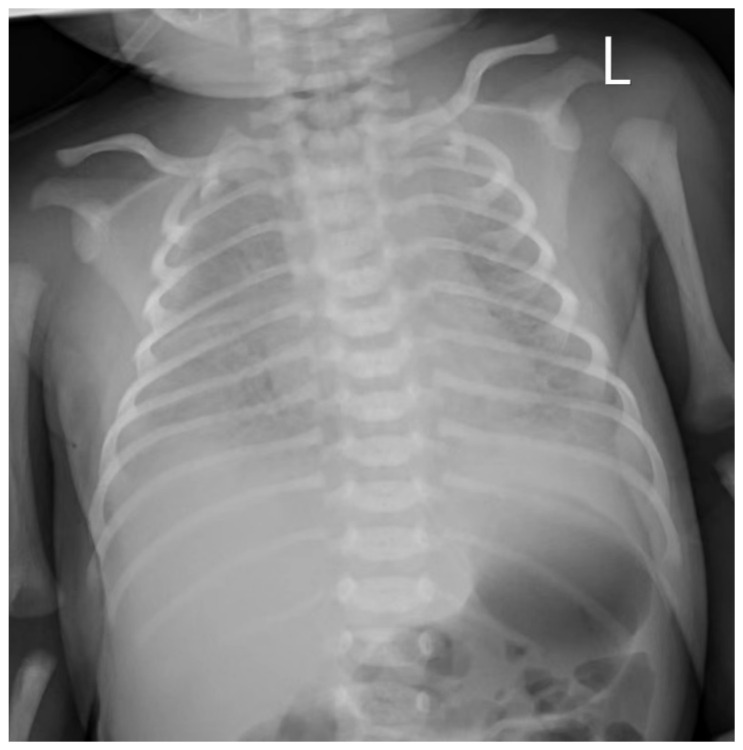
Chest radiography of neonates with GBS sepsis: significantly decreased bilateral transparency and extensive granular high-density shadows, with blurred bilateral diaphragmatic surfaces and costodiaphragmatic angles. L, left side of the neonate.
